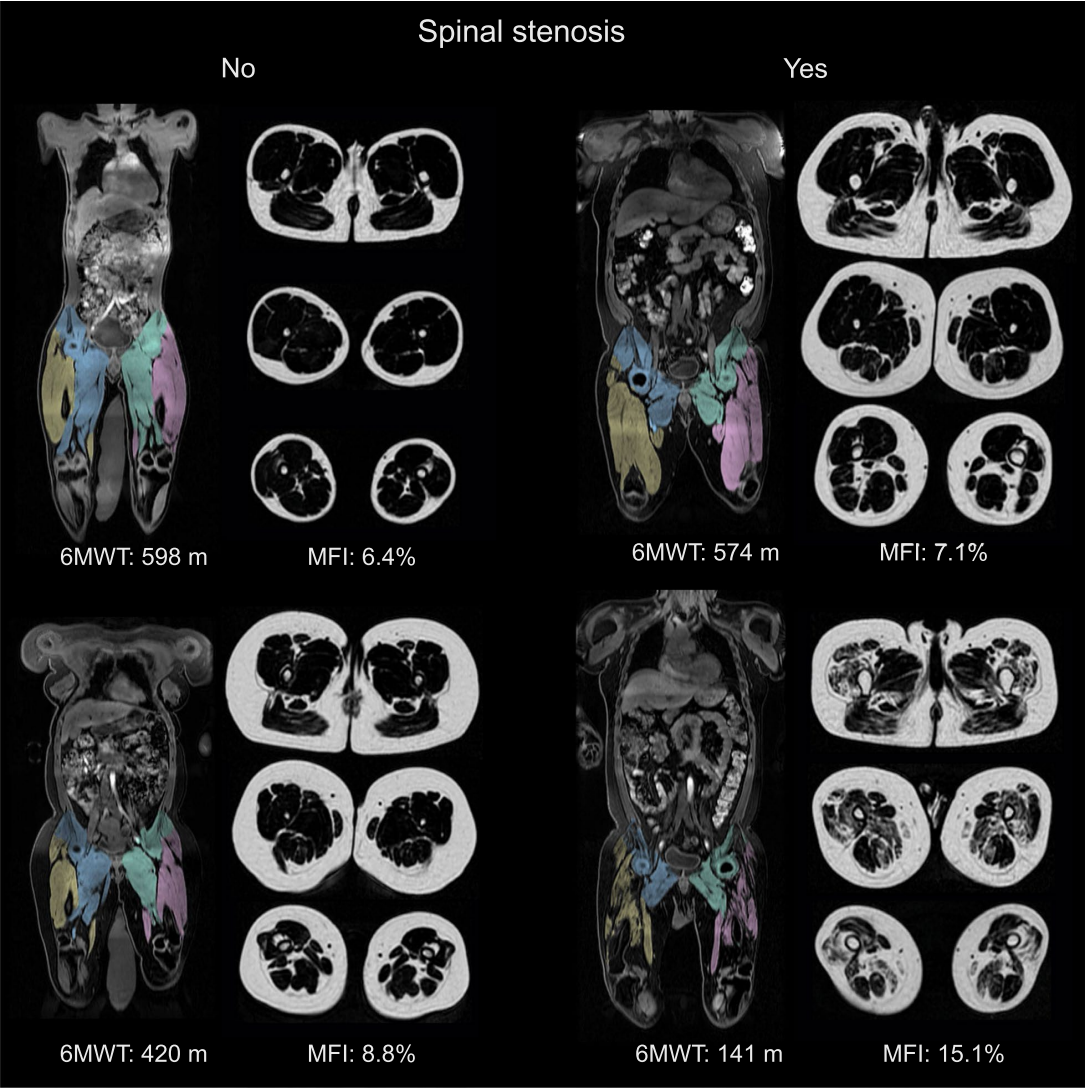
Examples of coronal and transverse magnetic resonance images in four adult participants with achondroplasia: two participants without spinal stenosis (to the left), and two participants with symptomatic spinal stenosis (to the right). The performance in the six-minute walk test (6MWT) and muscle fat infiltration (MFI) in the anterior compartment are provided for each of the participants. Muscle compartments are displayed in color
